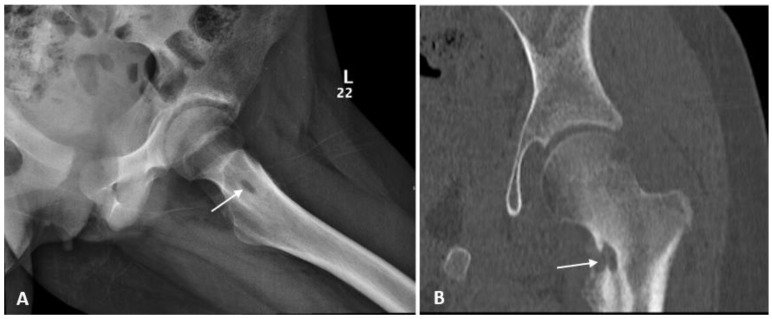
(A) Hip X-ray showed increased sclerosis with central lucency at the medial aspect of left femoral neck associated with the cortical irregularity and periosteal reaction; (B) Computed Tomography (CT) scan confirmed the erosive nature of the lesion with adjacent sclerosis and the adjacent bony overgrowth.
